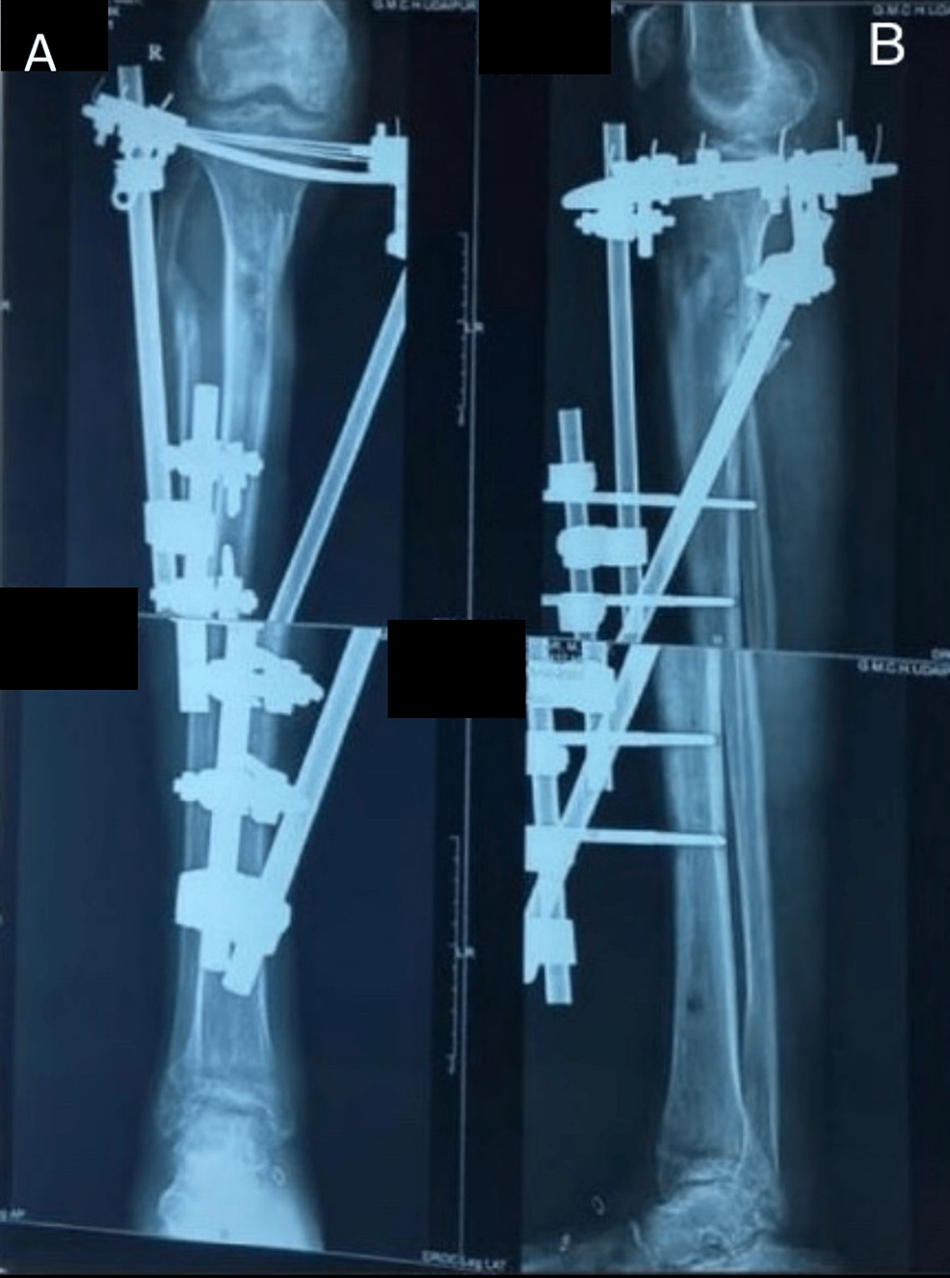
Sixth-month postoperative follow-up radiograph. Final follow-up radiograph showing fracture union. Figure A is an anteroposterior (AP) and Figure B is a lateral view.
